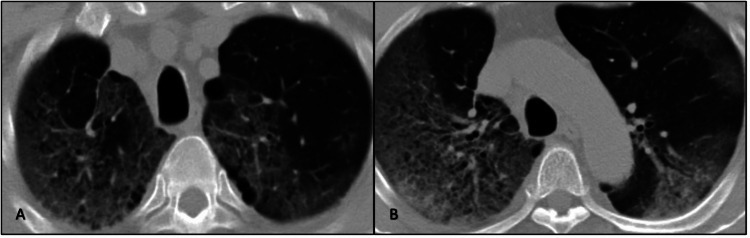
A and B: CT scan of the chest without contrast showing bilateral ground-glass opacities, more prominent and extensive on the right side, extending from upper to lower lobes. Additionally, patchy consolidations bilaterally with air bronchograms.
